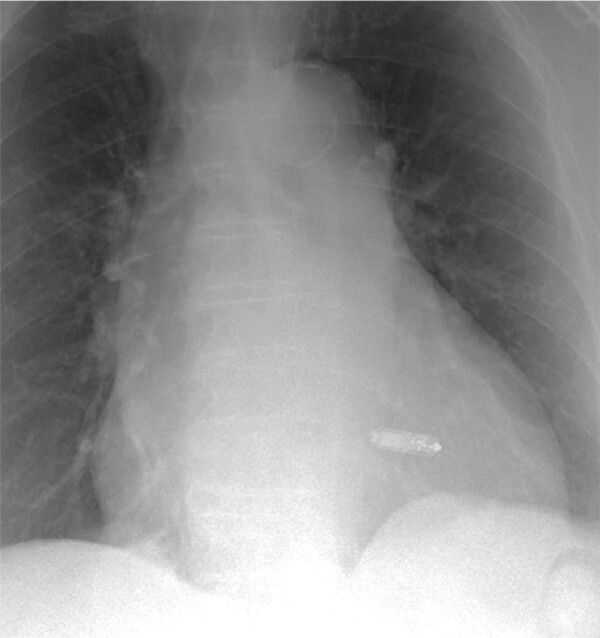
Case report 1. Chest X-ray—after complete removal of the stimulation system and leadless pacemaker (Medtronic Micra VR) implantation.
